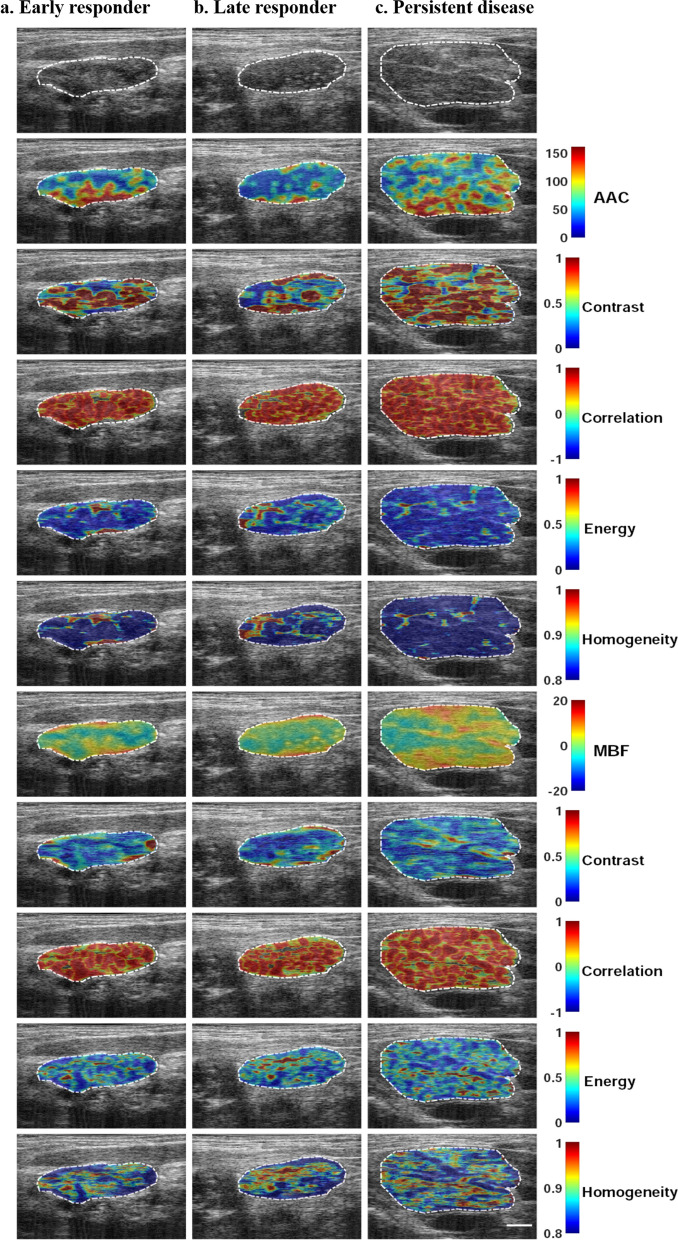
Representative ultrasound B-mode (uppermost row), QUS spectral parametric maps of AAC and MBF, and the corresponding texture images from one patient in each of the three response groups-early responder (a), late responder (b), and persistent disease (c). QUS parametric images include the largest involved lymph node (central region bounded by closed dotted white curve). The colour bar ranges are 0 to 150 dB/cm^3^ for AAC, − 20 to 20 dB for MBF and arbitrary unit for the texture features. The scale bar represents 1 cm.
